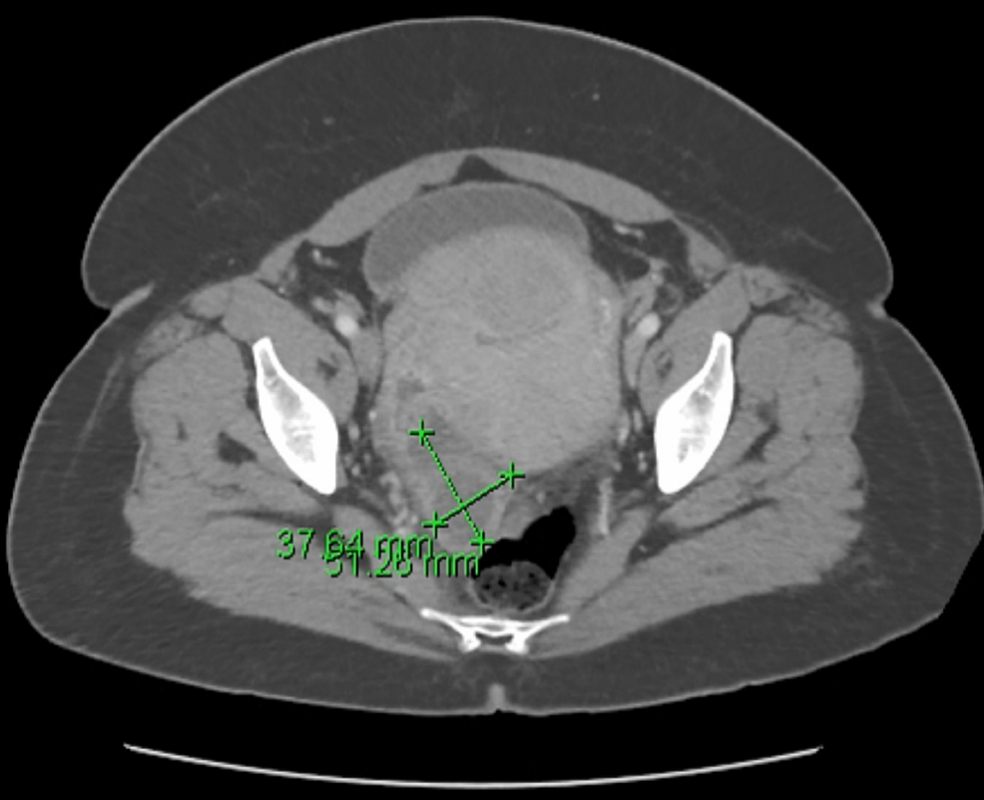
A repeat CT abdomen and pelvis shows slight interval enlargement of the fluid collection, now measuring 37.64 mm x 51.26 mm. CT: Computed tomography
